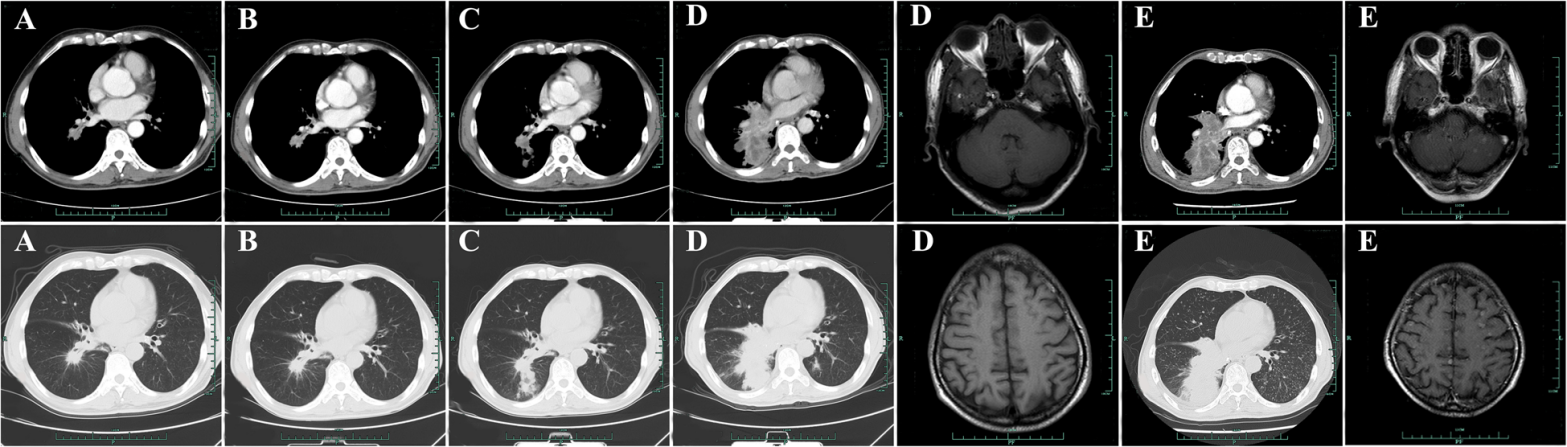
Chest CT scan and brain MRI from April 2016 to March 2017. a Chest CT scan after 1 month treatment of AZD9291 in April 2016. b Chest CT scan after 3 months treatment of AZD9291 in June 2016. c Chest CT scan after 6 months treatment of AZD9291 in September 2016. d Chest CT scan and brain MRI after 10 months treatment of AZD9291 in January 2017. e Chest CT scan and brain MRI after 2 cycles of docetaxel treatment in March 2017
